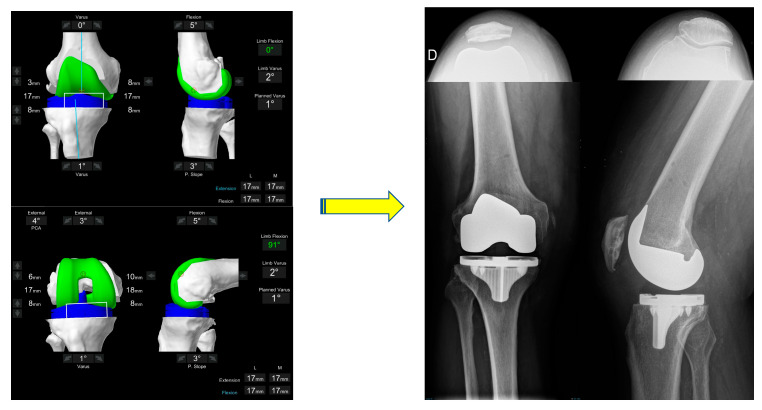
The final intra-operative position. Limb alignment, resections depth and tibiofemoral gaps in flexion and extension are shown. The achieved coronal alignment is 178°, or 2° varus and the sagittal alignment is 0° of flexion. The final gaps are 17 and 18mm, respectively. The post-operative X-ray at 3 months follow-up of the same patient is shown. The patella is well centred on the skyline X-ray.
